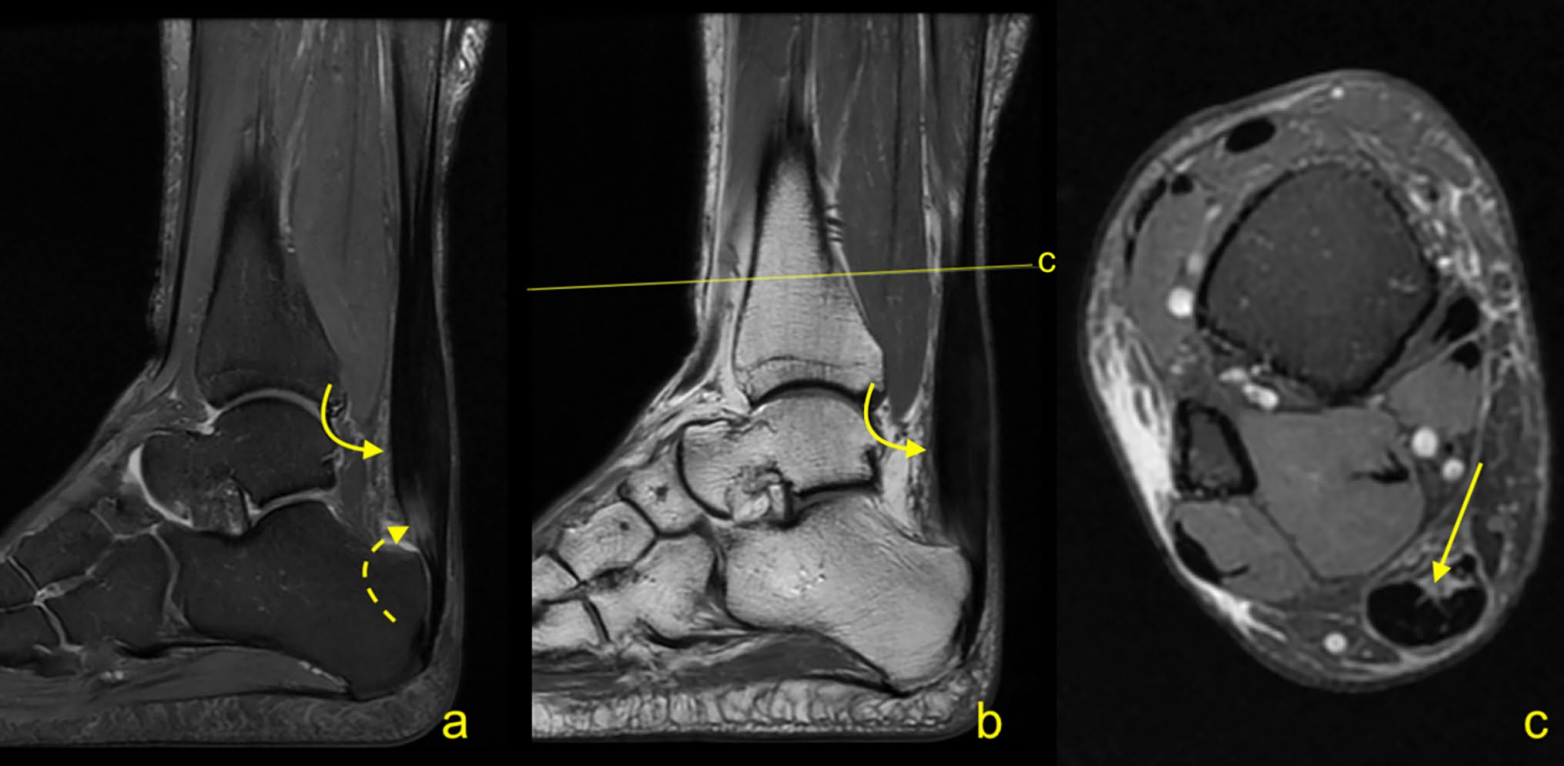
A 34-year-old male with painful Achilles tendinopathy and a long free tendon measuring 77.2 mm. MRI (a PD−w fat sat, b PD−w, c PD−w fat sat) revealed convex outline of the Achilles tendon (curved arrow) with high signal (curved dashed arrow). The lowest axial section (c) where the soleus muscle fibers can be identified (arrow)
